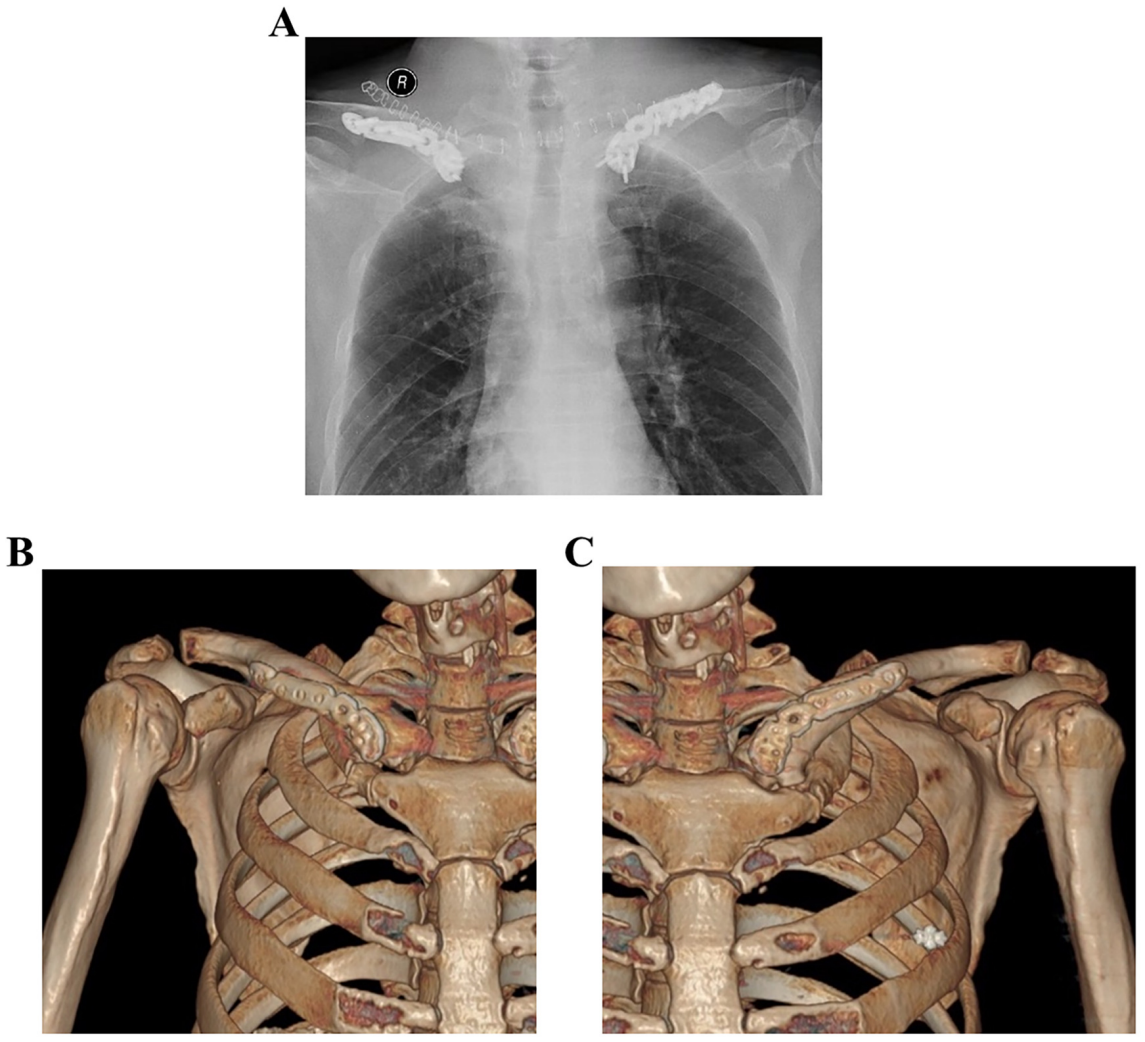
Postsurgical radiograph scans and CT scans. (A) Postsurgical radiograph demonstrating restoration of shoulder length after reduction and internal fixation with an inverted anatomic locking plate; (B,C) at eight months, CT scan revealed the union of both fractures.
